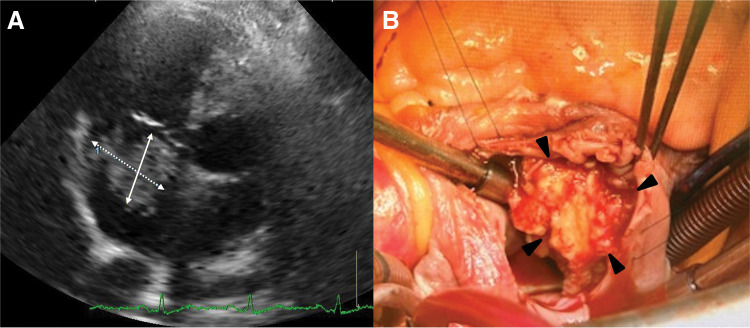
(A) Transthoracic echocardiography showing a 50 × 30 mm mass in the right atrium (arrows). (B) Intraoperative image showing tumor attached to the right atrial wall (arrow heads).
